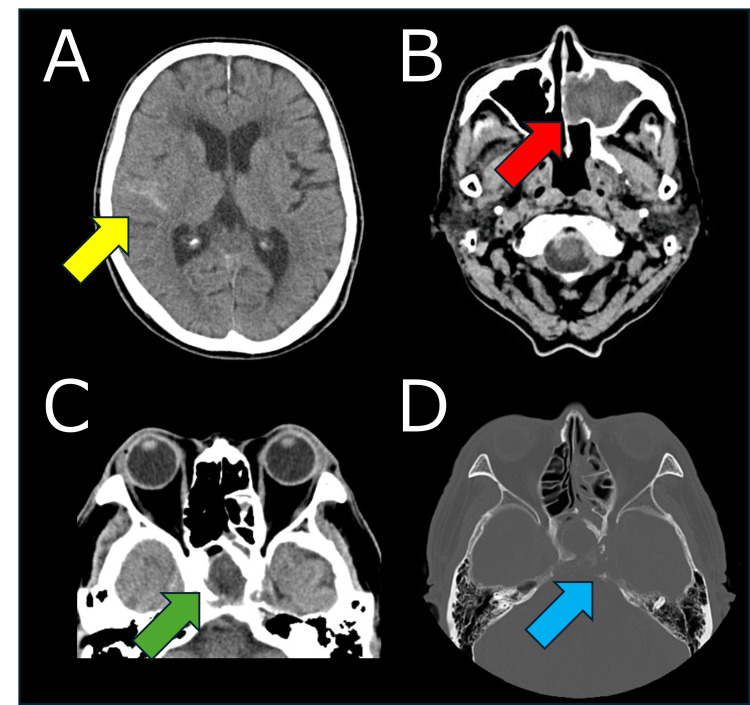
Brain CT at Admission. The initial CT revealed a hyperdense area in the right Sylvian fissure (A; yellow arrow) and a soft tissue shadow in the left maxillary (B; red arrow) and sphenoid (C; green arrow) sinuses, partially accompanied by osteolytic changes (D; blue arrow). CT: computed tomography.
